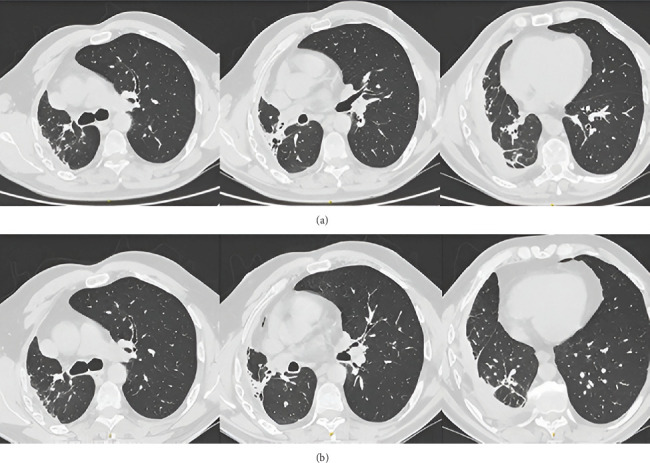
Follow-up CT scans performed on the (a) 7th of June 2023 and (b) 21st of February 2024—Case 2.
